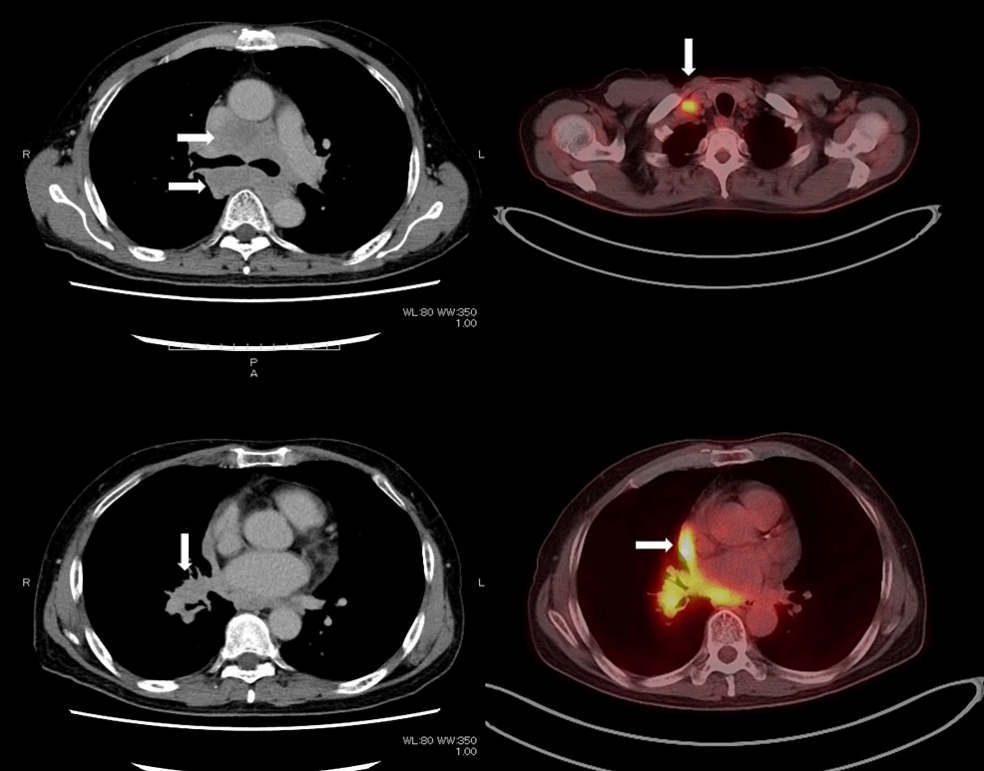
Imaging findings at the initial visit. Computed tomography (CT) scan at the initial visit showing a large mass consisting of mediastinal lymph node swelling (white arrows, top left) and a tumor in the right lower lobe (white arrow, bottom left). Positron emission tomography-CT also revealed positive lymph node metastasis in the right subclavian (white arrow, top right), as well as positive findings of cancerous pericarditis (white arrow, bottom right).
